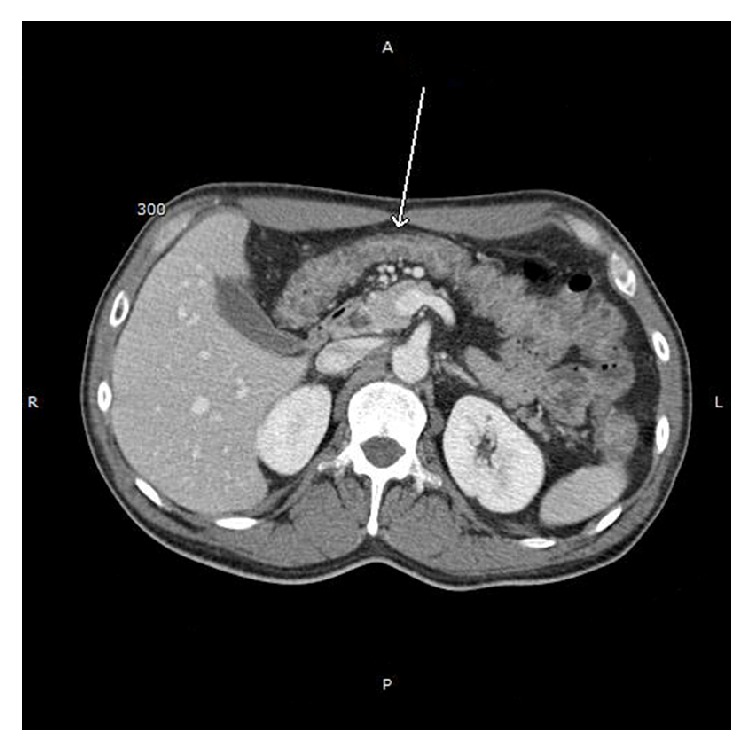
Abdominal computed tomography showing diffuse circumferential thickening of the colonic wall. The arrows refer to circumferential thickening of the transverse colon.
